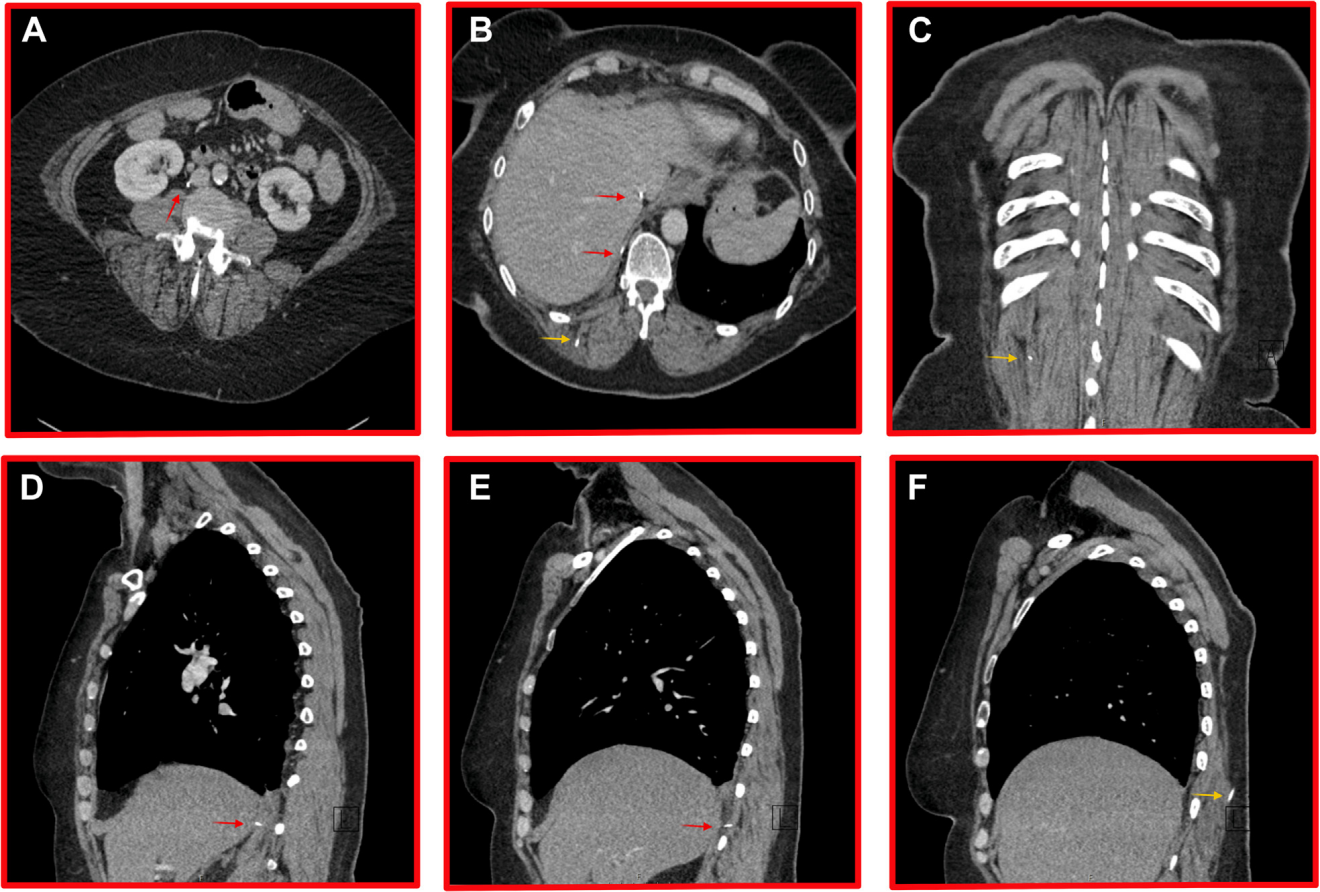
Posterior Course of the Wire, With 6 Separate Still Images Taken From Computed Tomography of Different Slices In each respective view, the red arrow details the cause of the wire within the abdomen, and the yellow arrow details the extravascular course within muscle or subcutaneous tissue. (A) Transverse thorax slice. The wire is seen exiting the heart posteriorly into the posterior basal segment of the right lower lobe. The exit point is thought to be the junction of the inferior vena cava and the right atrium. (B) Transverse thorax slice. The wire has gone beneath the diaphragm. (C) Coronal thorax slice. The exit point is via the right paravertebral soft tissues adjacent to T12. (D) Sagittal thorax slice. This view shows the wire traveling posteriorly. (E) Sagittal thorax slice. The wire is seen exiting adjacent to T12. (F) Sagittal thorax slice. The wire is seen traveling through the soft tissues to just beneath the skin.
